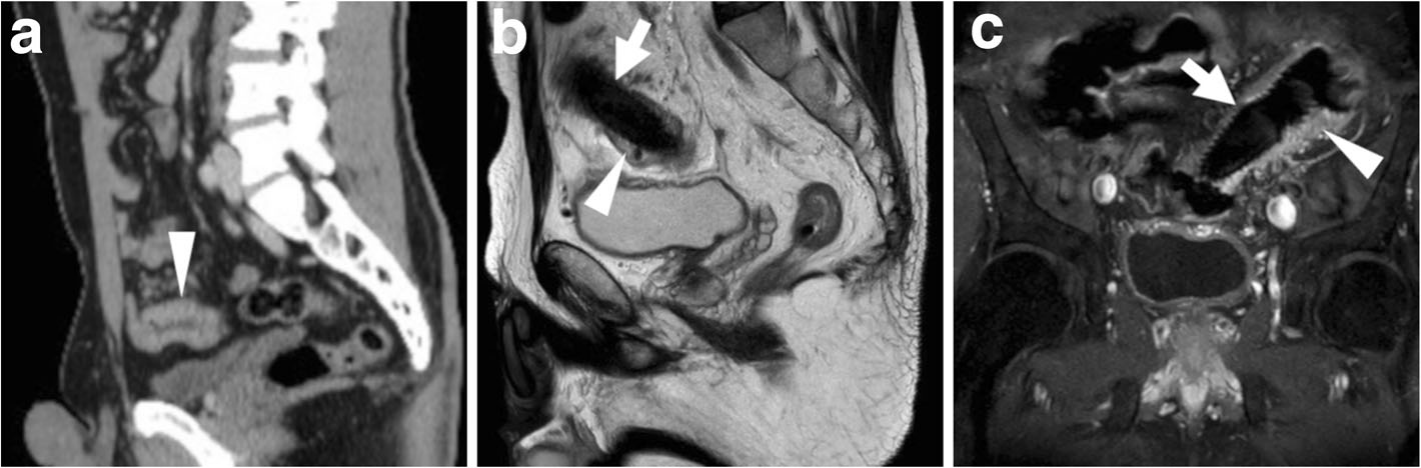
Usual MRI appearance of a SEMS-treated CRC. At diagnosis, sagittal CT image (a) shows an endoscopically impassable, ulcerated CRC of the sigmoid colon as segmental mural thickening (arrowhead). After stent placement, sagittal T2- (b) and coronal fat-saturated T1-weighted after intravenous gadolinium contrast (c) MRI images show well-expanded SEMS (thick arrows) with patent lumen devoid of signal, and mural thickening (arrowheads) with T2-hypersignal (b) and positive enhancement (b) corresponding to the treated tumour
